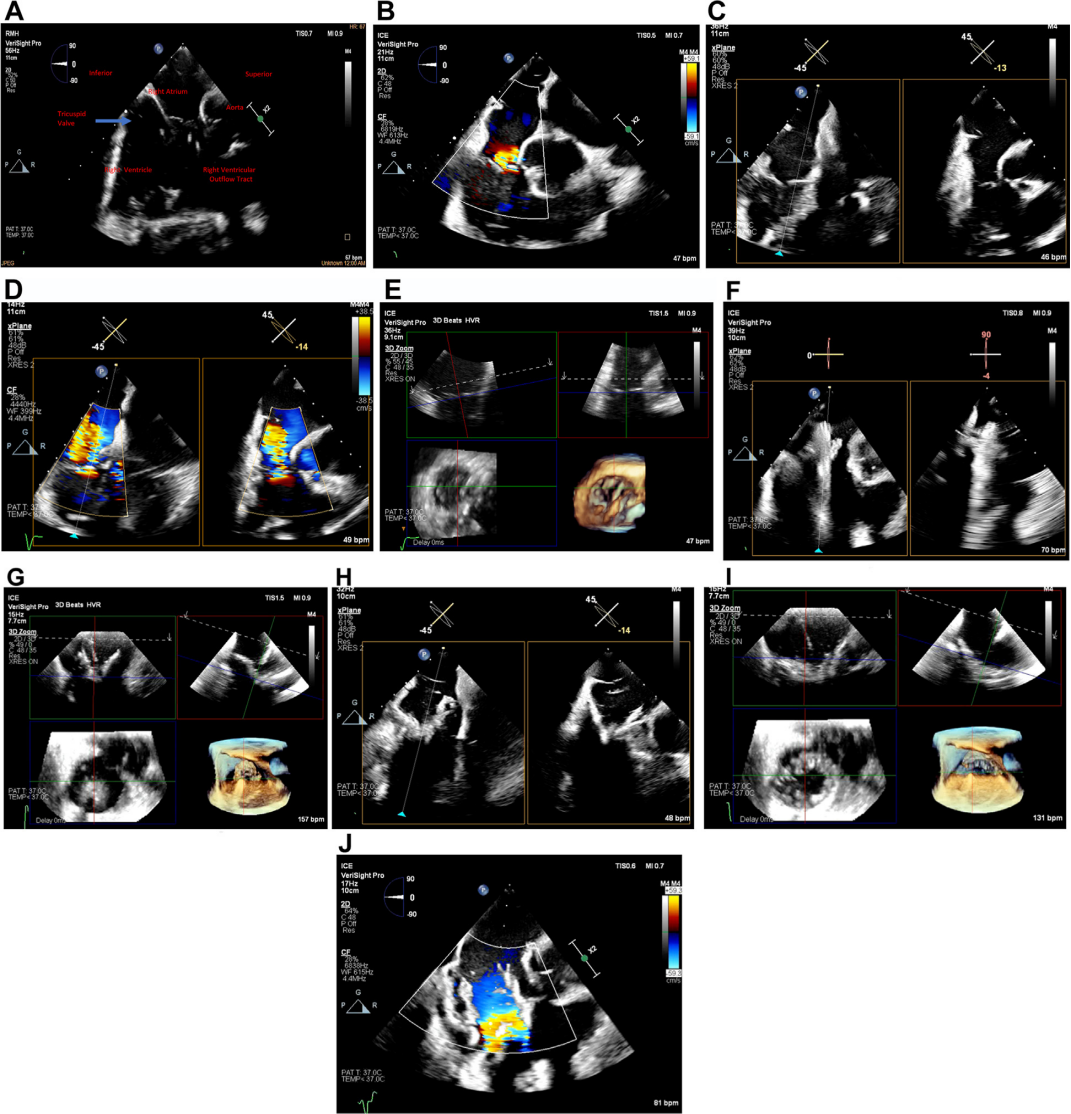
Intracardiac echocardiography imaging for transcatheter tricuspid valve replacement. (A) 2-dimensional ICE view showing all 3 tricuspid leaflets from home view without and (B) with color. (C) Right ventricular inflow/outflow biplane view without and (D) with color. (E) MPR view is obtained by placing the crosshair markers across the TV annulus, creating a 3D en face view of the TV in real time. (F) The TV is crossed with the capsule centered on biplane view. (G) The TTVR brim is opened above the TV, aligned above the TV parallel with the tricuspid plane, and centered within the annulus on MPR imaging. (H) The TTVR is advanced once the position is properly aligned with the annulus. Biplane imaging confirms the brim of the bioprosthesis is coaxially aligned to the TV annulus and (I) MPR imaging is used to confirm proper alignment of the bioprosthesis in multiple orthogonal views. (J) The TTVR is deployed on a biplane view once positioning is confirmed. Images were obtained using the Philips VeriSight PRO 3D ICE catheter. 3D, 3-dimensional; ICE, intracardiac echocardiography; MPR, multiplane reconstruction; TTVR, transcatheter tricuspid valve replacement; TV, tricuspid valve.
